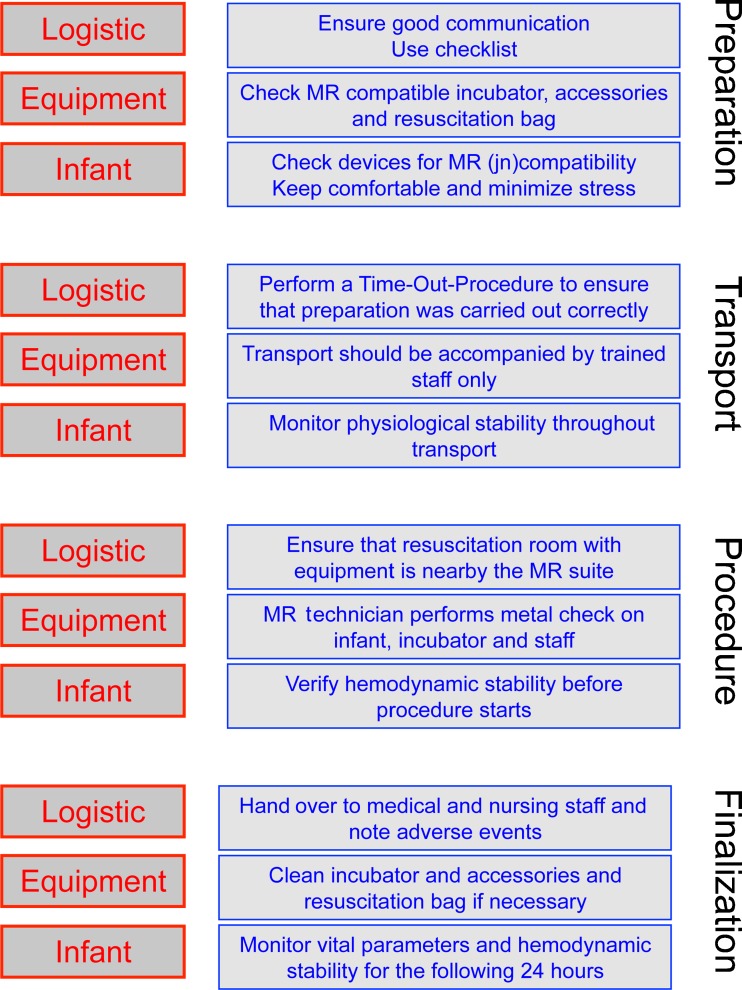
Guideline for safe execution of MRI procedures in preterm infants (see Online Resource 1)
